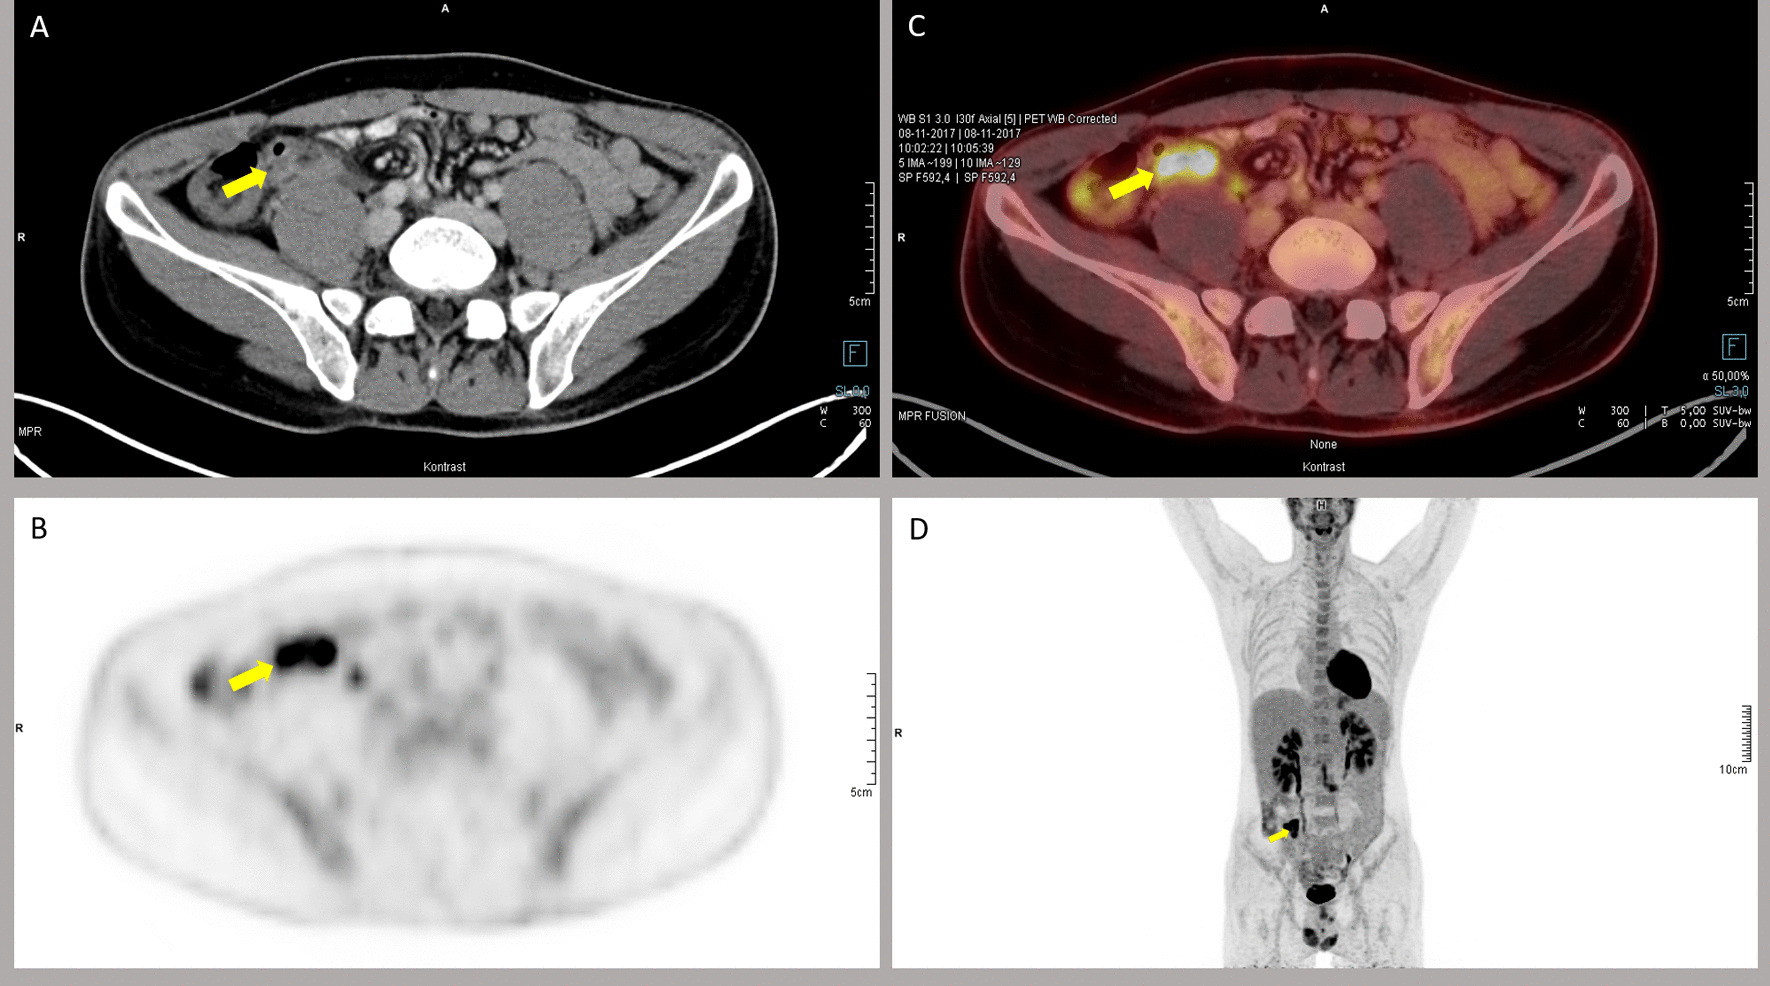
Patient 2. ^18^F-FDG PET/CT. A CT-scan with intravenous and per oral contrast (transaxial plane) of the abdomen at the level of the appendix showing increased diameter of the appendix. B
